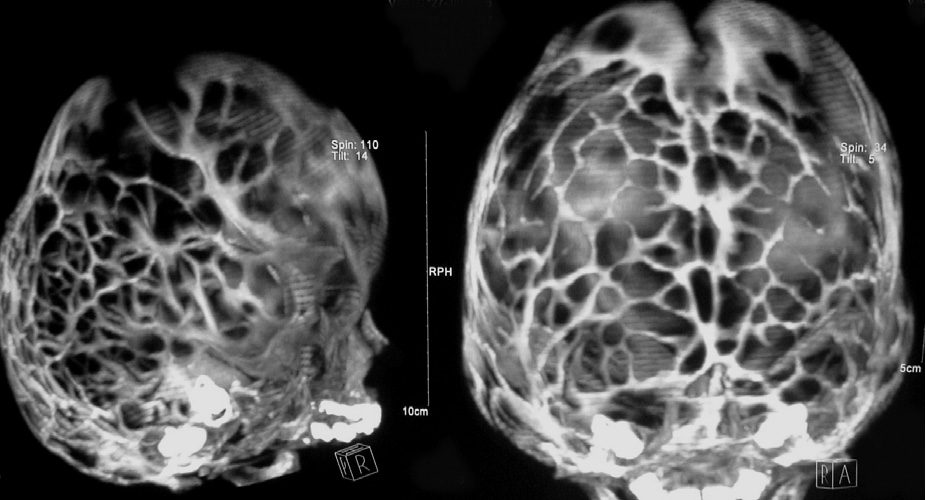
Tri-dimensional computed tomography scan confirmed the fusion in the coronal and lambdoid sutures and diffuse skull changes with honeycomb configuration and beaten copper appearance.
